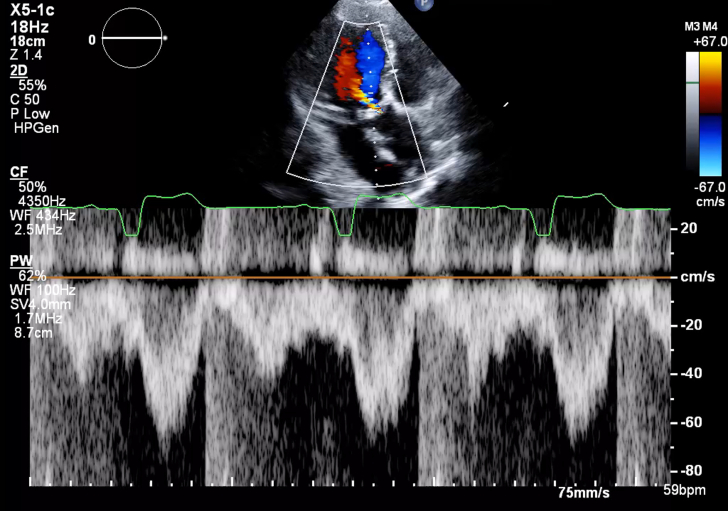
Apical 5-Chamber View Pulse Wave Doppler Assessment Apical 3 chamber: align PW sample volume in the left ventricular outflow tract avoiding the area of flow convergence.
